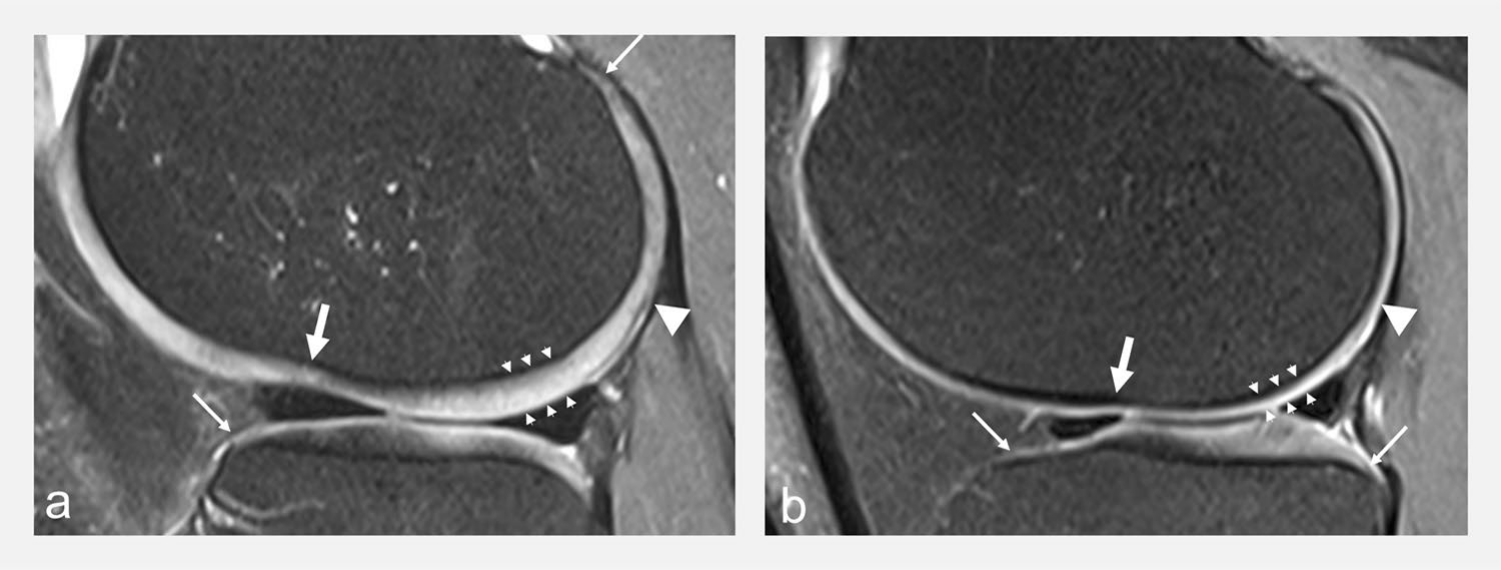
Sagittal FS IW MR images at the level of the lateral femoral condyle in a a 46-year-old asymptomatic male volunteer and b a 35-year-old asymptomatic female volunteer showing a normal inter-individual variation of cartilage thickness (between arrowheads), and physiological areas of cartilage thinning overlying the lateral femoral condylar notch (white arrows), the posterior lateral femoral condyle (arrowheads), and at the margins of cartilage (thin arrows)
